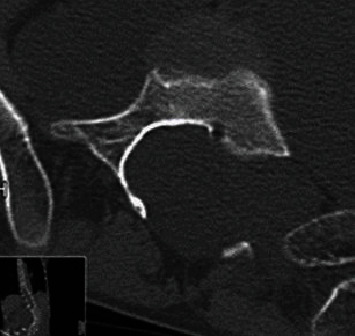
Preoperative axial CT. Dural ectasia and massive intraperitoneal myelomeningocele.
